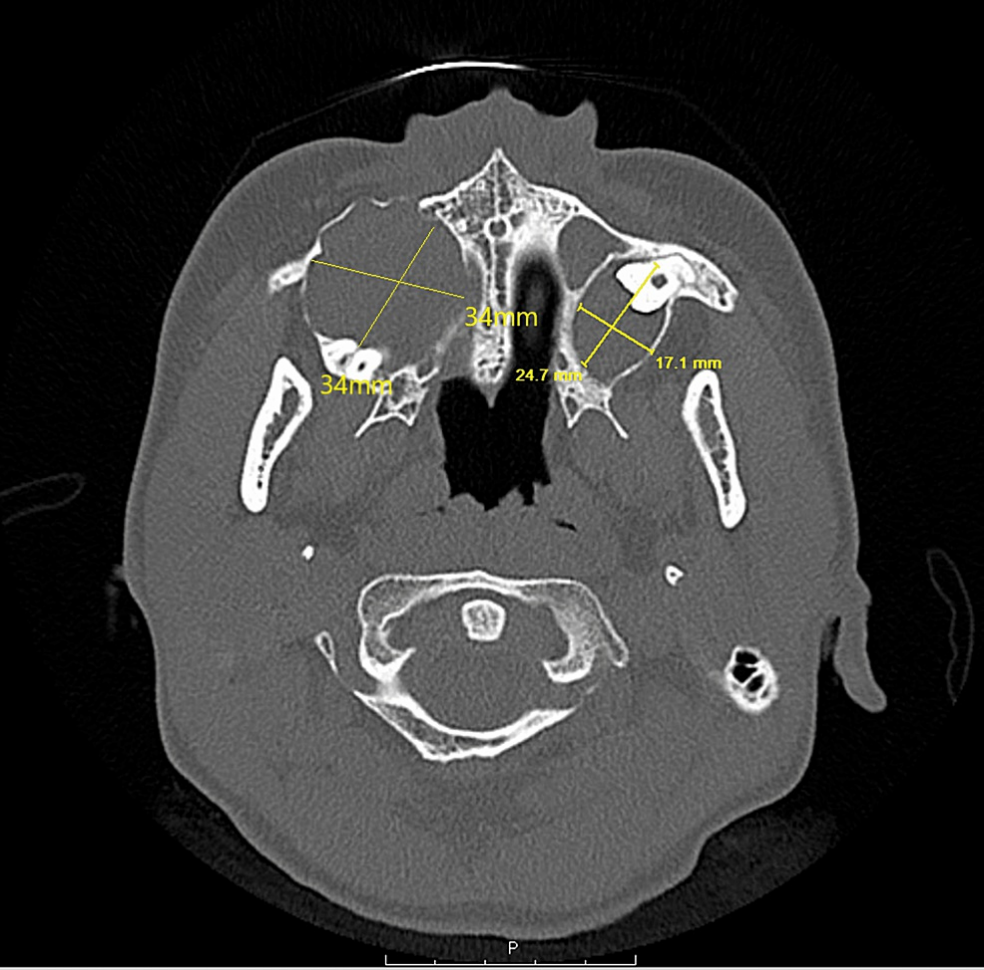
CT maxillofacial w/o contrast There is a 1.7 cm × 2.4 cm left-sided dentigerous cyst with expansion of the posterior maxillary wall and 3.4 cm × 3.4 cm dentigerous cyst of the right maxillary sinus with expansion thinning of the surrounding maxillary sinus walls.
